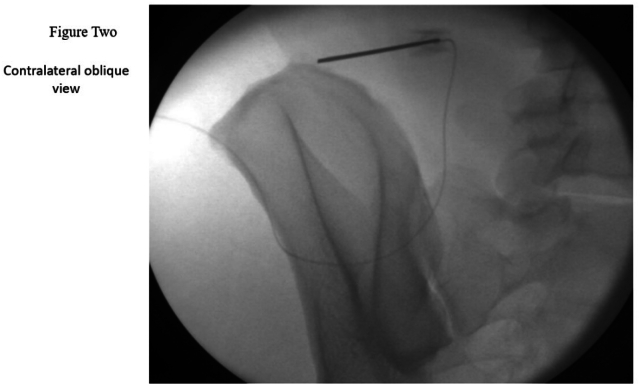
Xray Contralateral oblique view; *The introducer is shown in a contralateral oblique view, with its tip positioned above the iliac crest.*
